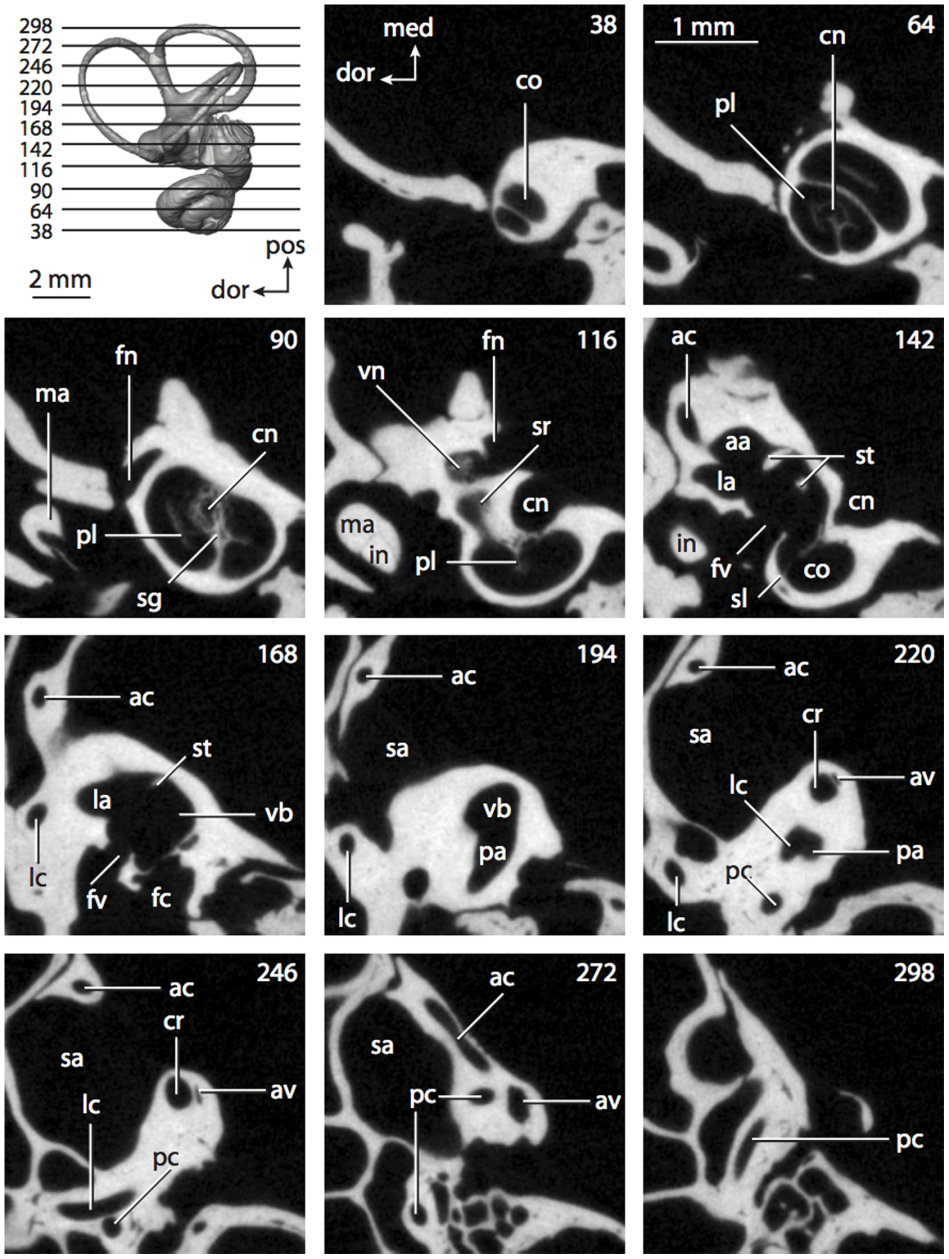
CT slices through ear region of*Cynocephalus volans*. Abbreviations listed at the end of the Materials and Methods section.
